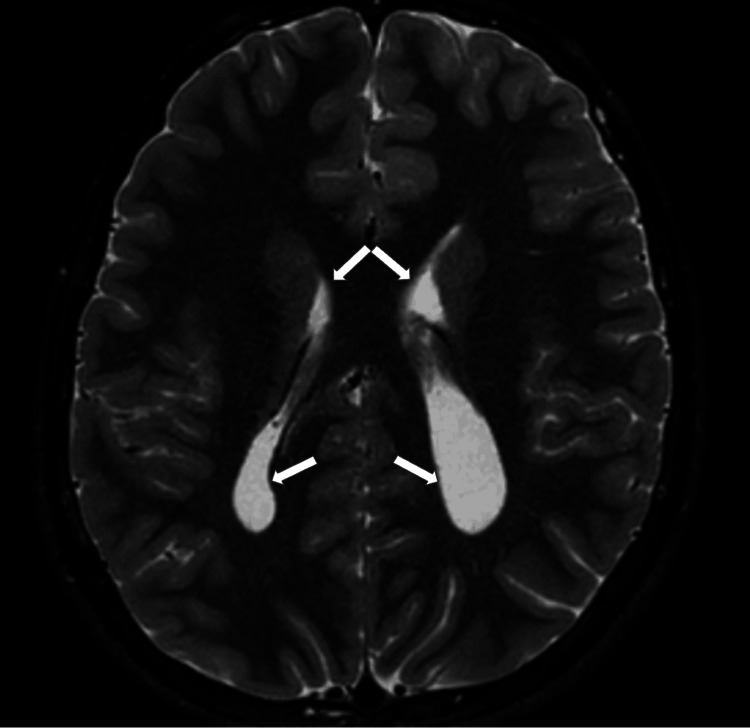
Axial T2-weighted MRI of the brain without contrast shows colpocephaly with mild ventriculomegaly (white arrows) T2 - transverse relaxation time
